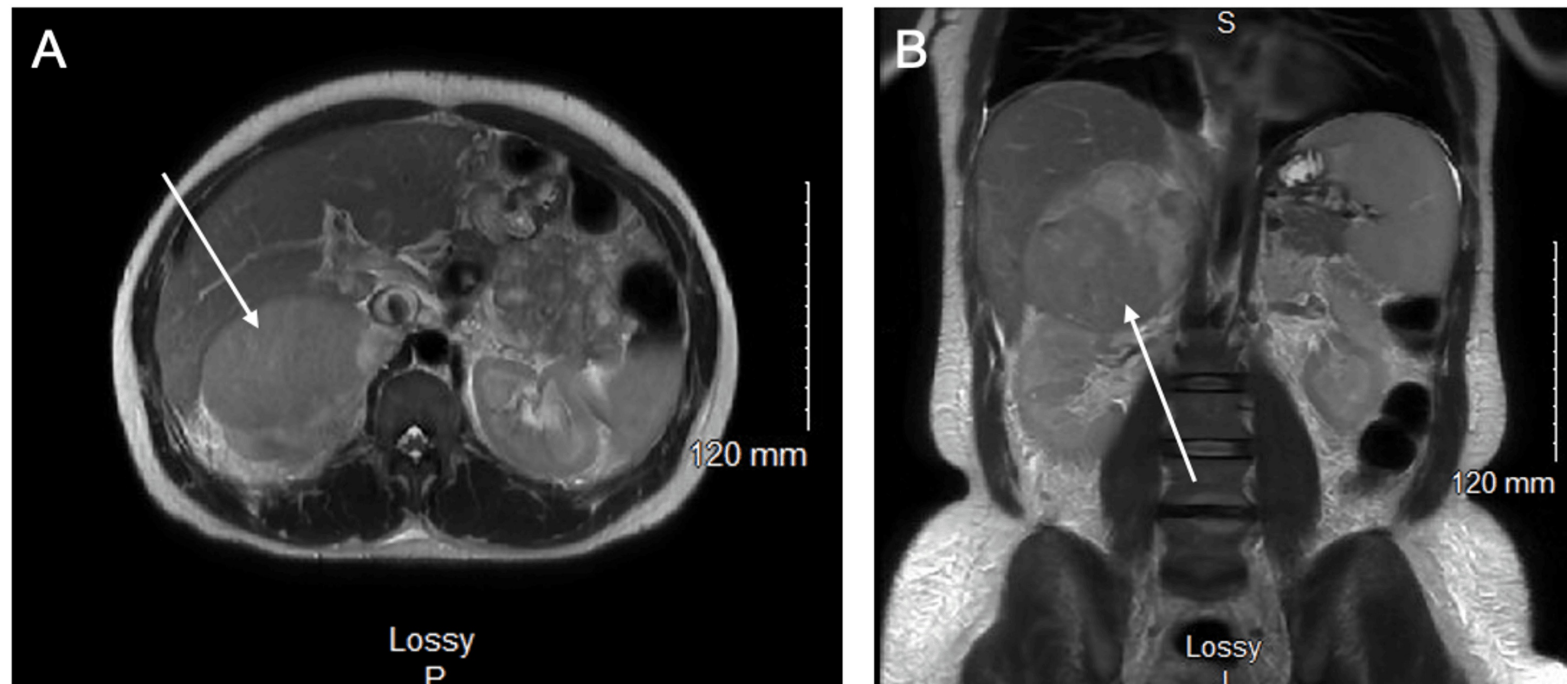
MRI indicating a right adrenal mass. (A) Axial MRI shows an abnormal adrenal mass with mass effect on the posterior right hepatic lobe. (B) Coronal MRI shows the same adrenal mass.
